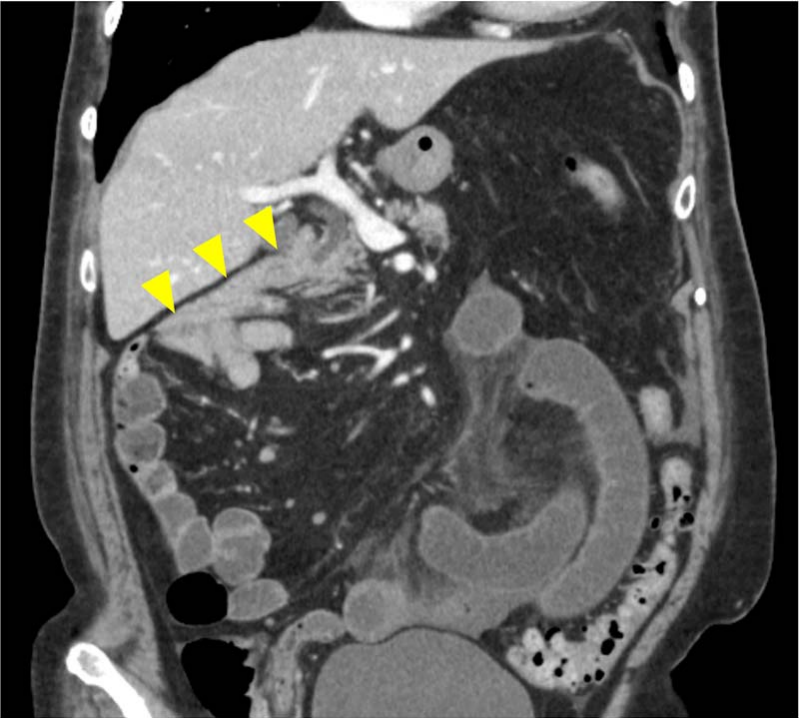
Image from enhanced CT. Contrast-enhanced CT shows the absence of a horizontal duodenal leg and the duodenum running caudally to the jejunum (arrowhead).
